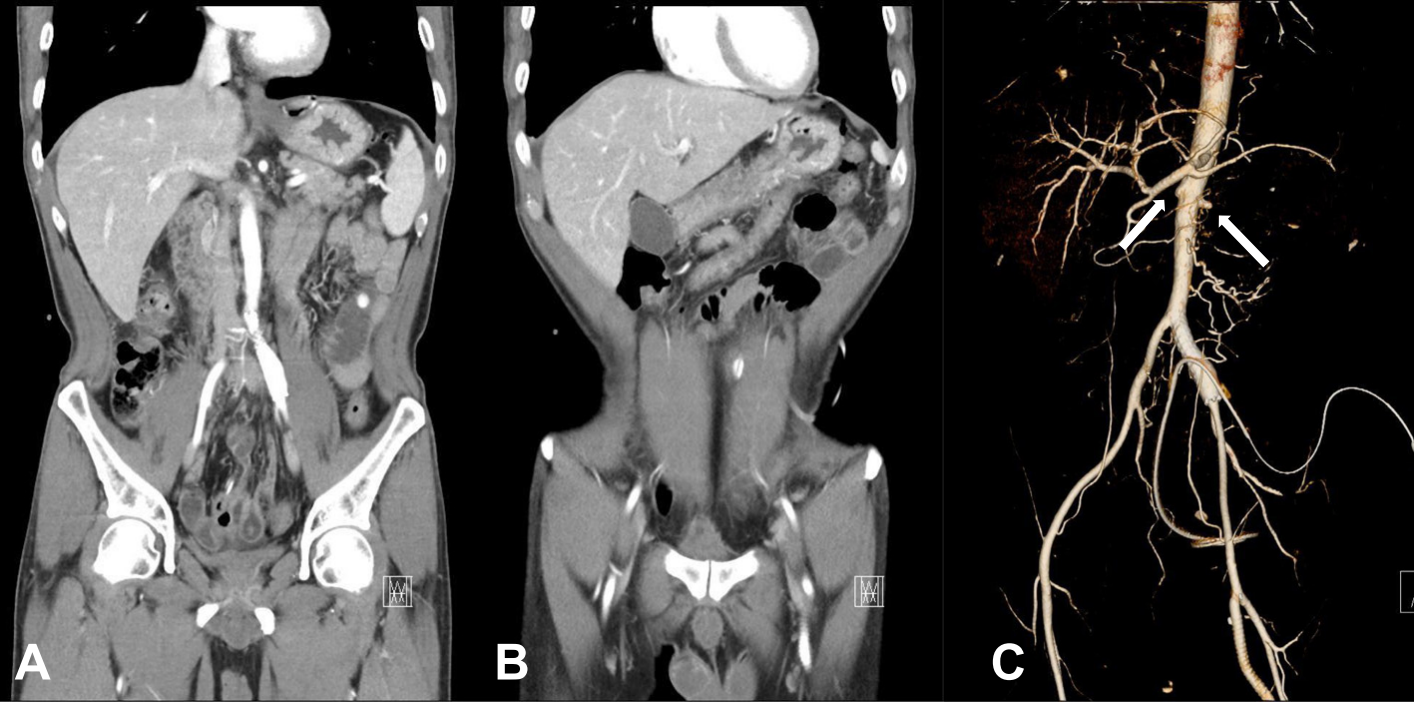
Contrast-enhanced abdominal CT and abdominal aorta CT angiography. Contrast-enhanced abdominal CT demonstrated colitis of the (a) hepatic flexure and (b) transverse colon, most likely due to ischemic colitis. c Abdominal aorta CT angiography showed total occlusion of both renal arteries (*white arrows*). Superior and inferior mesenteric arteries cannot be seen because the arteries were occluded from their origins
